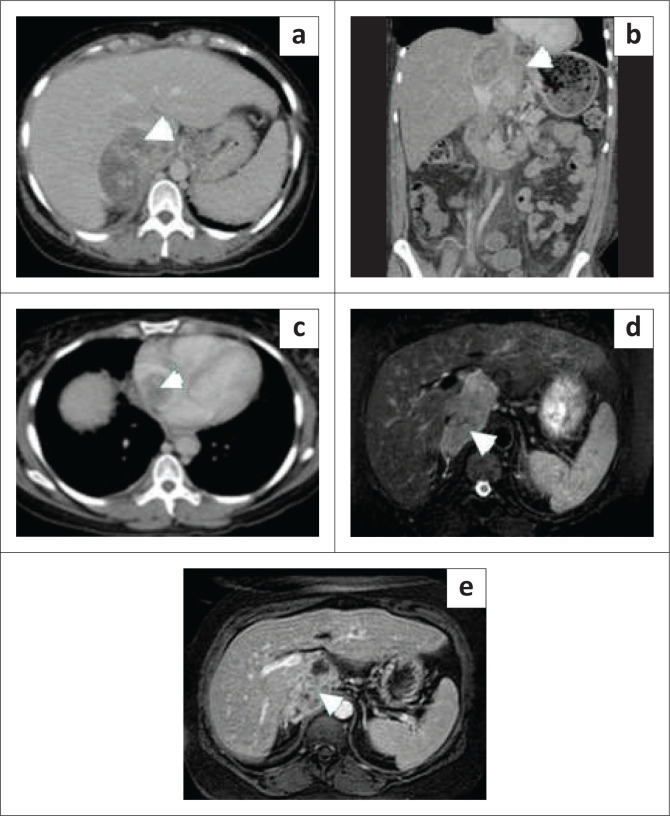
Inferior vena cava (IVC) leiomyosarcoma. (a) Contrast-enhanced axial CT abdomen showed a heterogeneous enhancing mass (arrowhead) expanding the IVC lumen. (b) The coronal contrast-enhanced CT image showed the mass (arrowhead) has a significant exophytic component in the retroperitoneum. (c) The axial CT image demonstrated extension of the mass (arrowhead) into the right atrium. (d) The axial T2-weighted fat saturated image showed a slightly T2 hyperintense mass (arrowhead) expanding the IVC lumen. (e) The post-contrast axial fat saturated image showed a heterogeneously enhancing mass (arrowhead) in the IVC lumen.
